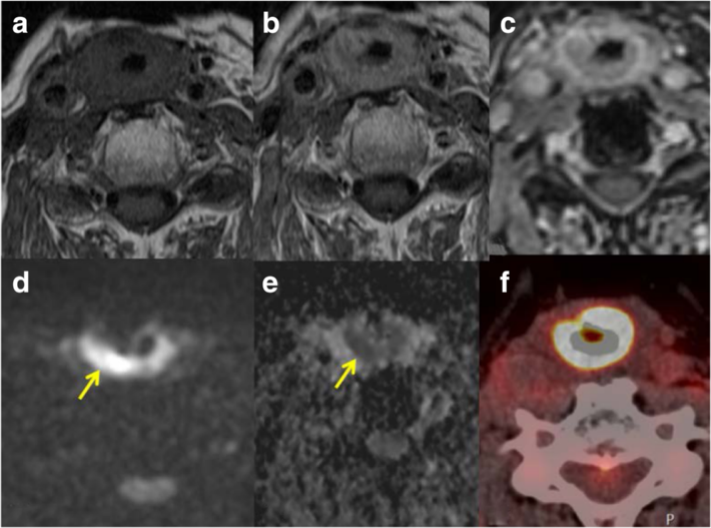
Axial T1-weighted MR image before contrast a T1-weighted MR image after injection of gadolinium without fat saturation b and with fat saturation c in a patient with diffuse thickening of the neopharynx (radial free flap reconstruction) after total laryngectomy for larynx cancer. DW-MRI shows hyperintense signal (arrow) in the b800 image d with corresponding low signal in the ADC map e indicating tumour recurrence. The PET-CT image f showed intense uptake of glucose (see also Fig. 10) over a long segment due to which it was interpreted as inflammation
